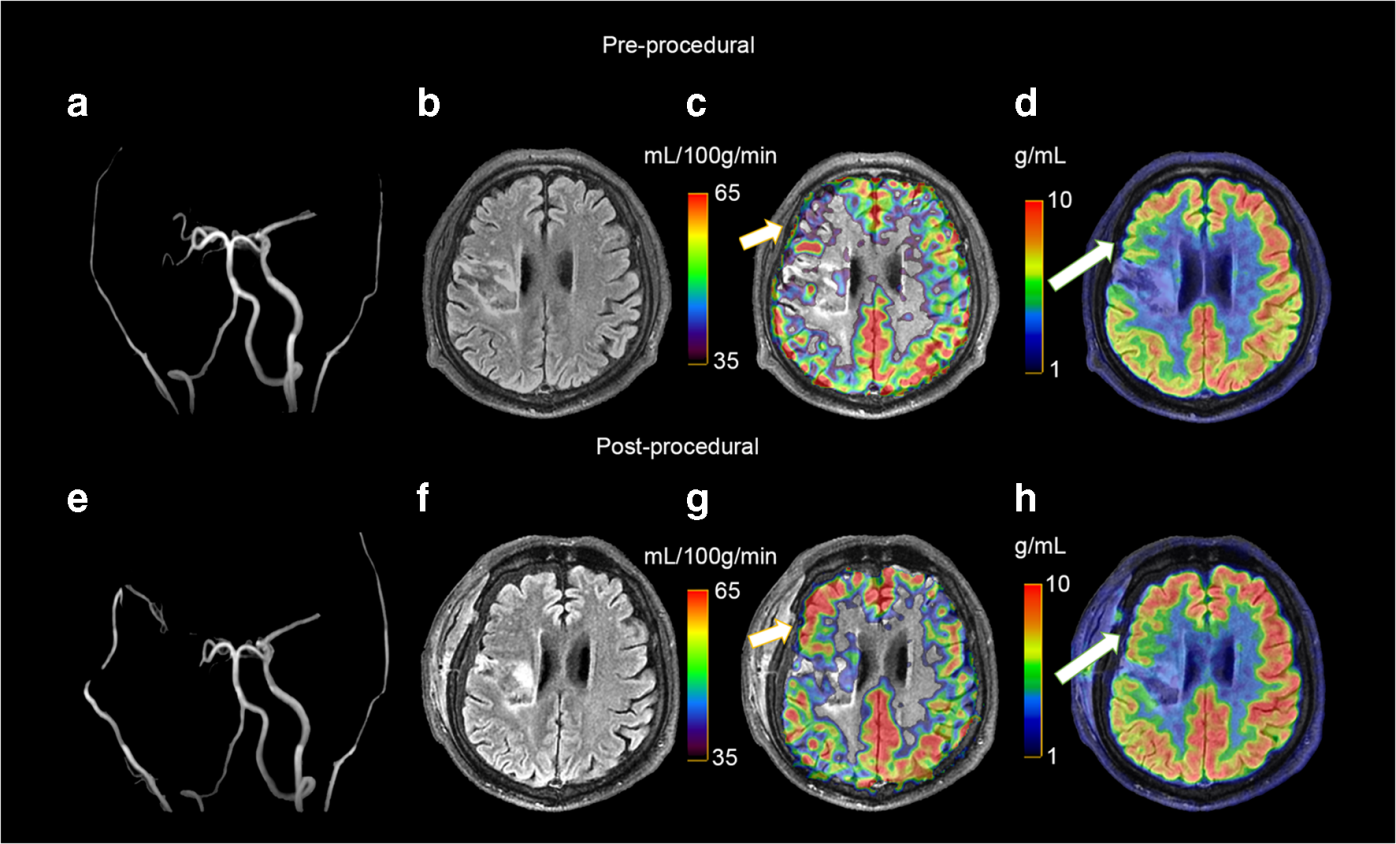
Representative preprocedural and postprocedural images obtained in a 51-year-old man with symptomatic occlusion of the right ICA who underwent bypass surgery. Preoperative MRA (a) showed severe ICA occlusion, and postoperative MRA (e) showed an end-to-side anastomosis between the STA and MCA. MRA T2 FLAIR images (b and f) showed infarction in the left radial crown and corpus callosum. Postprocedural fused CBF/MR image (g) showed improvement compared to the preprocedural image (c) on the affected side (short arrow). Postprocedural fused ^18^F-FDG PET/MR image (h) showed improvement compared to the preprocedural image (d) on the ipsilateral side (long arrows)
